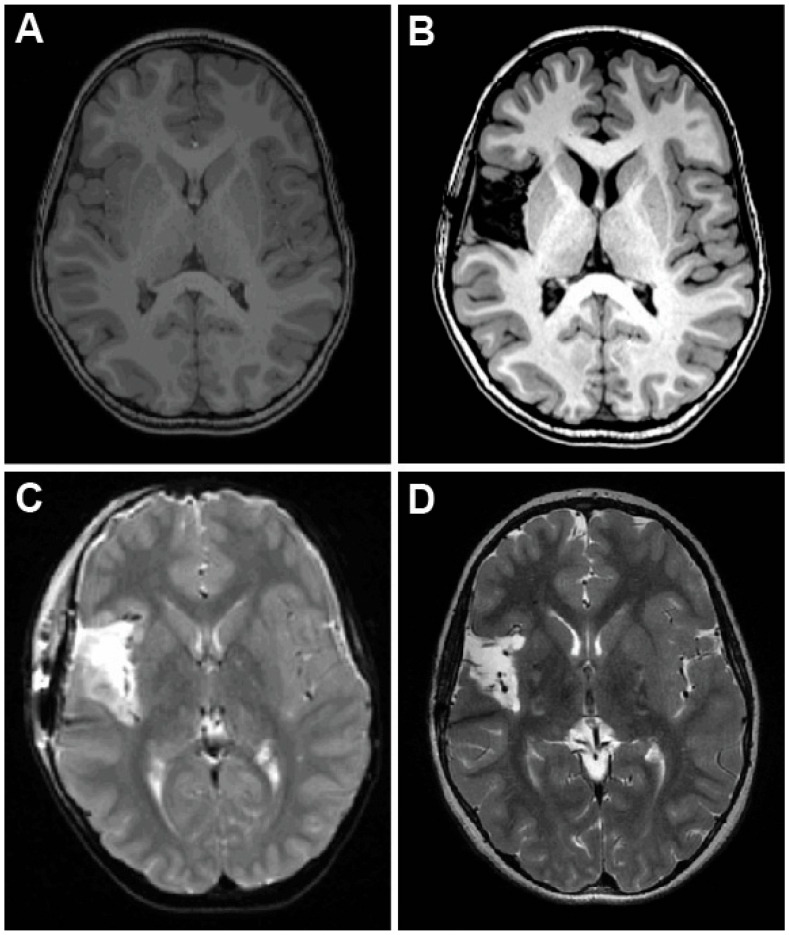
Pre- and postoperative MRI for Case 4 demonstrating complete insular resection. (A) Preoperative T1-weighted axial MRI demonstrating no focal anatomic abnormality in the right insular region. (B) Postoperative axial T1-weighted MRI demonstrating near-complete insulectomy. (C) Immediate postoperative axial T2-weighted MRI demonstrating cytotoxic edema in corona radiata and adjacent perisylvian frontal and temporal lobes, which resolved by 2 months postoperative MRI (D).
